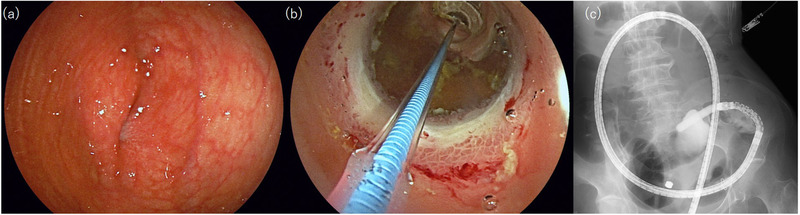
Endoscopic image: the stenotic lesion of the ileum before dilation using a balloon catheter (a). Endoscopic (b) and radiographic images (c) the stenotic lesion was dilated using a balloon catheter
